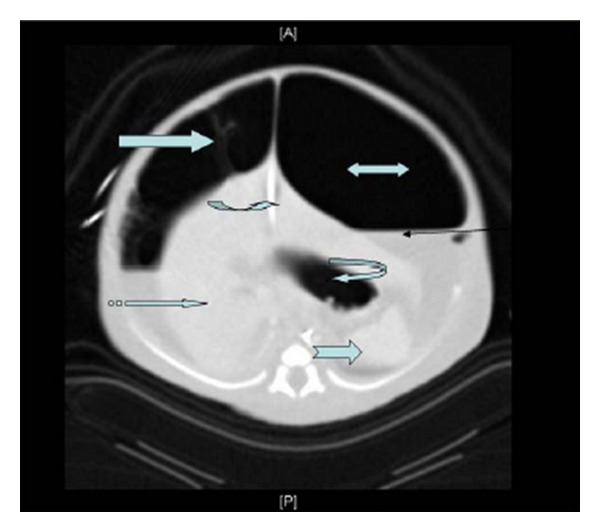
Abdominal CT scan image: showing abundant intraperitoneal space (biheaded block arrow) and fluid levels (black line arrow) with multiple thick septation (right block arrow) noted mainly at the right hypochondrium. Umbilical vein catheter (curved right arrow), spleen (notched right block arrow), stomach (curved left arrow), and liver (striped right block arrow) were noted.
